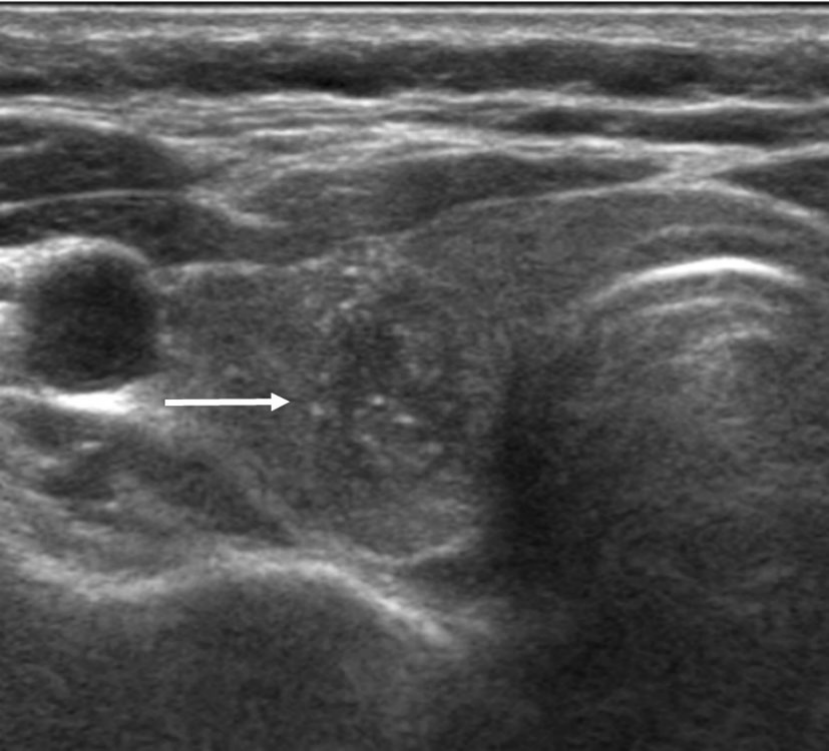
Asymptomatic anaplastic thyroid carcinoma in a 52-year-old woman. A transverse sonogram shows a 1.5-cm nonparallel oriented hypoechoic nodule (arrow) with echogenic dots in the right thyroid gland, indicating K-TIRADS 5.
